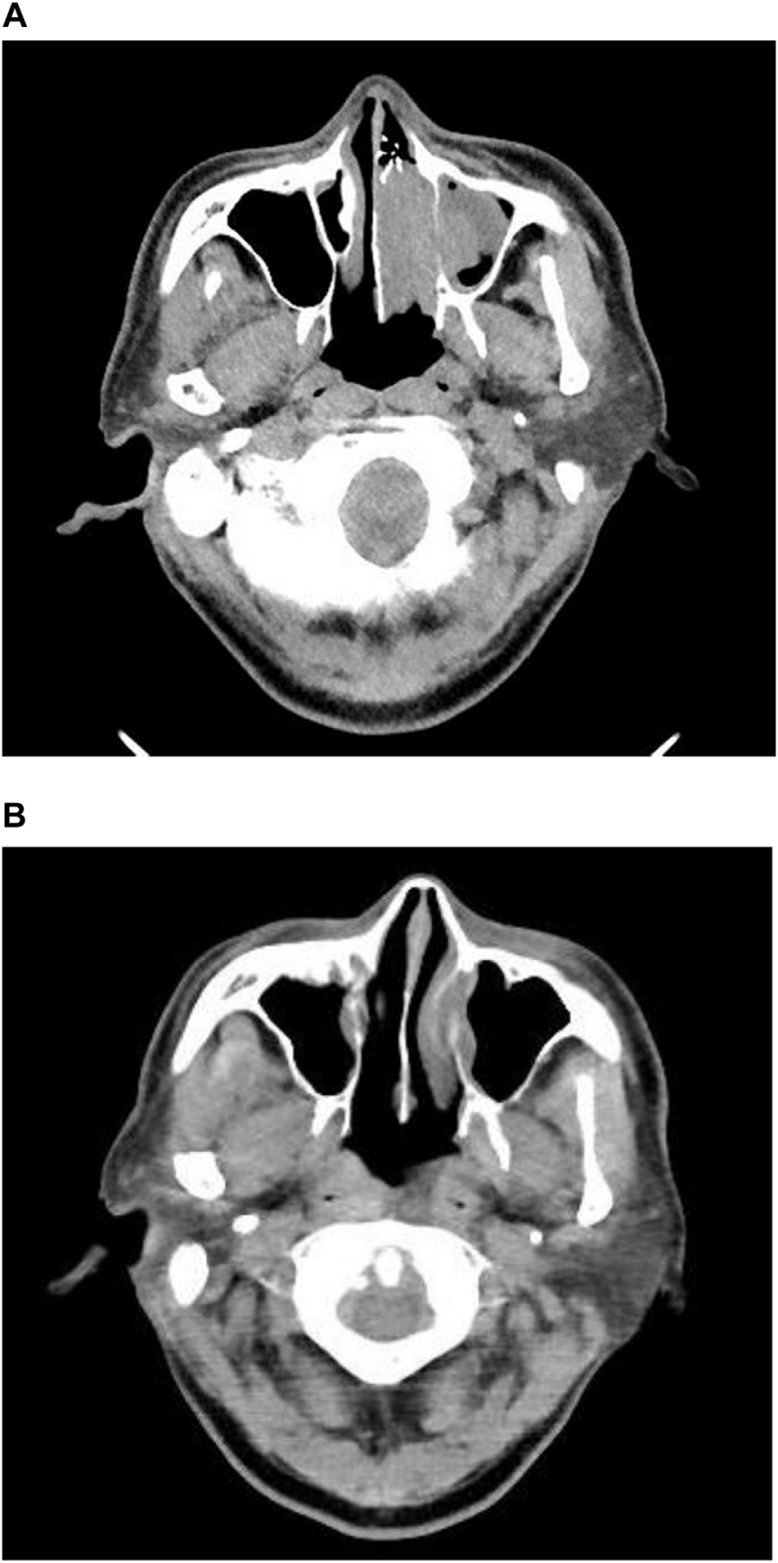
Contrast computed tomography at the first visit and after radiotherapy and chemotherapy (A). First visit: The left nasal concha was obstructed by the tumor. (B) Tumor remission after radiotherapy followed by nivolumab and ipilimumab therapy.
